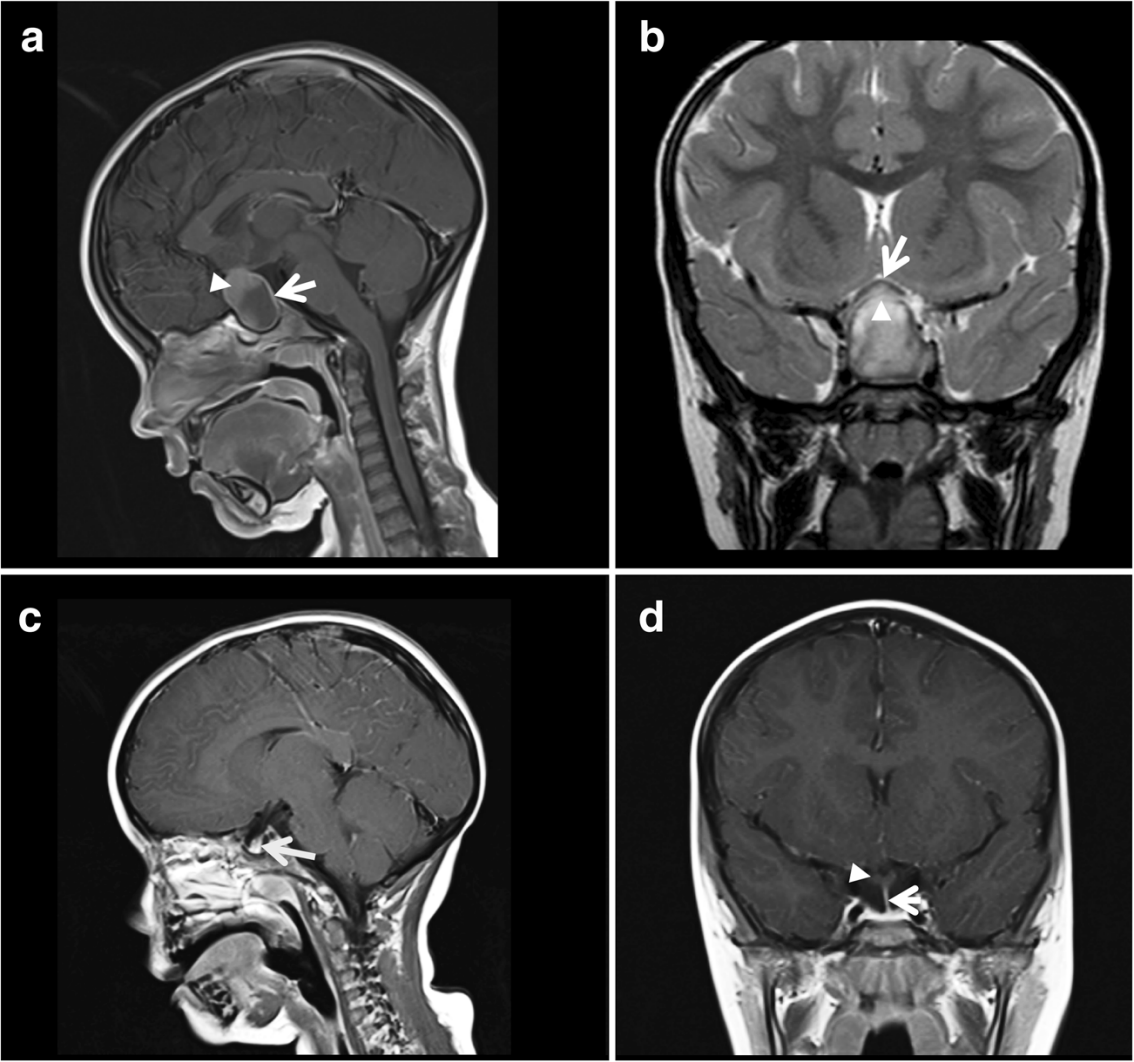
Sagittal pre-operative post-contrast T1 MRI brain scan image at presentation (a) revealing a large suprasellar lesion, measuring 3 × 1.9 × 2.3 cm. The lesion compromised a superior, solid component which had increased T1 signal pre-contrast (solid arrow head). This component also demonstrated high T2/FLAIR signal. An inferior, low FLAIR signal cystic component was noted. Peripheral rim enhancement of the lesion can be appreciated (white open arrow). b is a coronal T2 image also obtained at presentation which shows the upwards extend of the lesion (solid arrow) impinging onto and splaying the optic chiasm (open white arrow). Following transsphenoidal tumor surgery, follow-up sagittal T1 post-contrast MRI imaging (c) demonstrates improved appearances, with a small degree of non-enhancing residual tissue extending down into an enlarged pituitary sella (white arrow). The coronal T1 post-contrast image (d) now demonstrates a normal position of the optic chiasm (solid arrow) and a clearly discernable pituitary stalk which is slightly deviated to the left (white open arrow)
